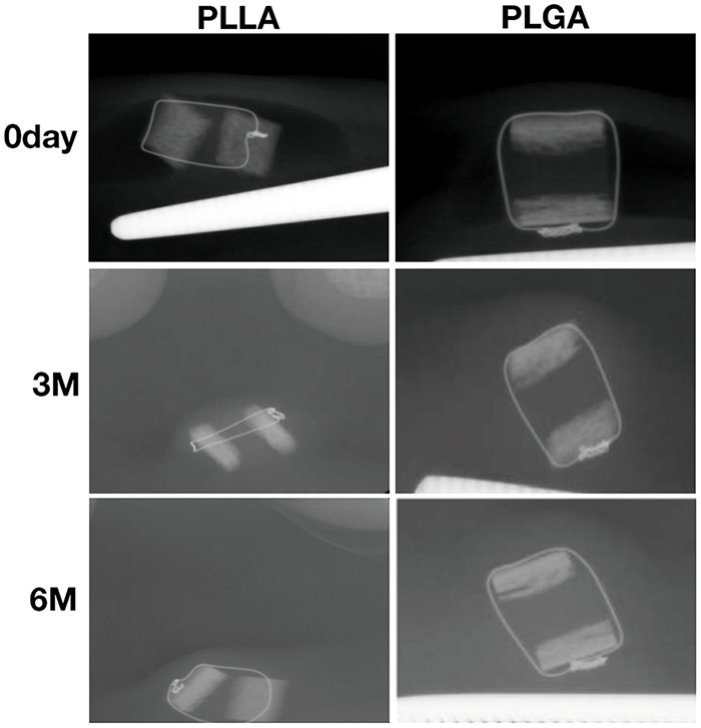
Findings of X-ray in PLLA/PLGA scaffolds with chondrocyte cells interposed with iliac bones. No calcification was observed in each sample.
